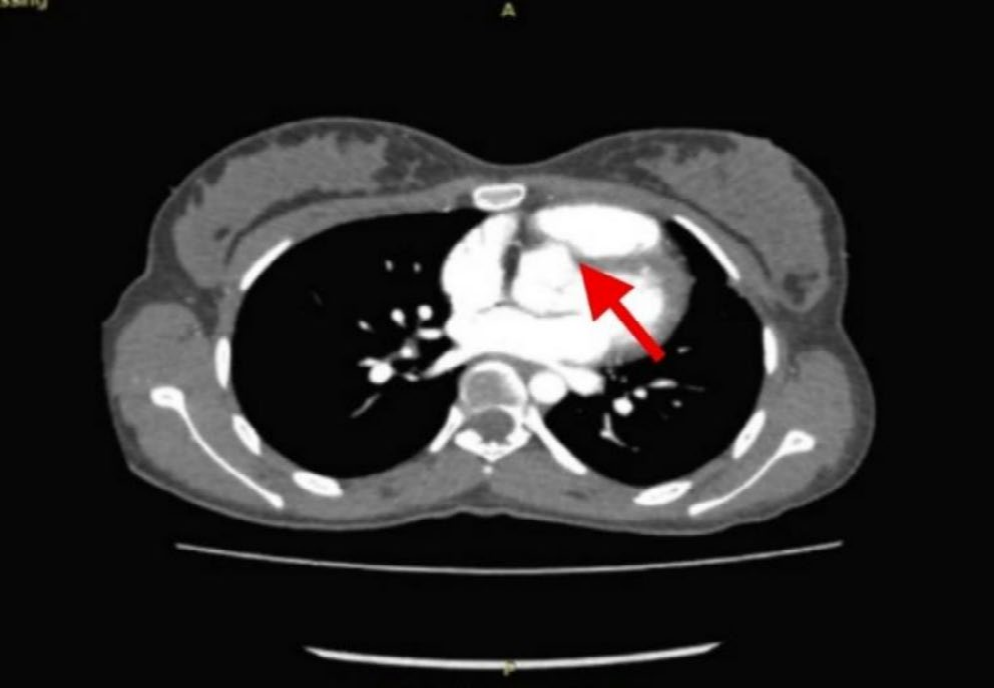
CT Scan showing communication between the right coronary cusp and RVOT.
